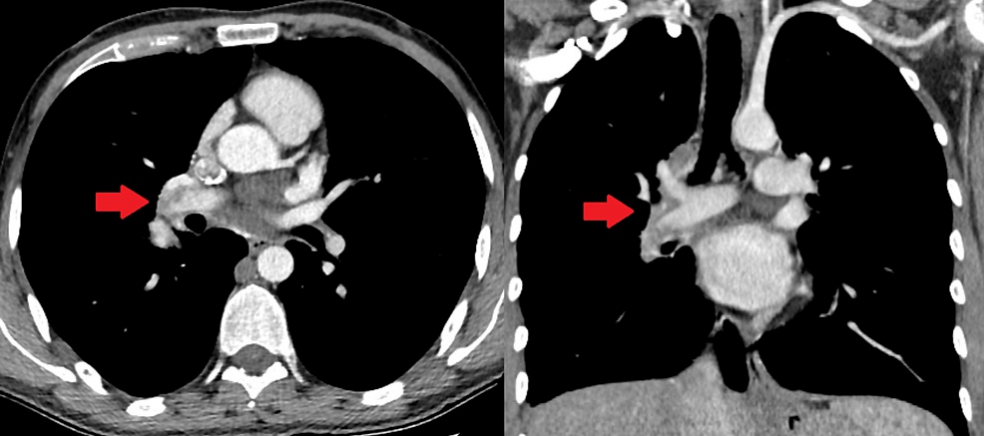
Treatment evaluation with CT chest Regression in both aneurysmatic lesions and pulmonary embolism was seen after four months of treatment CT: computed tomography
